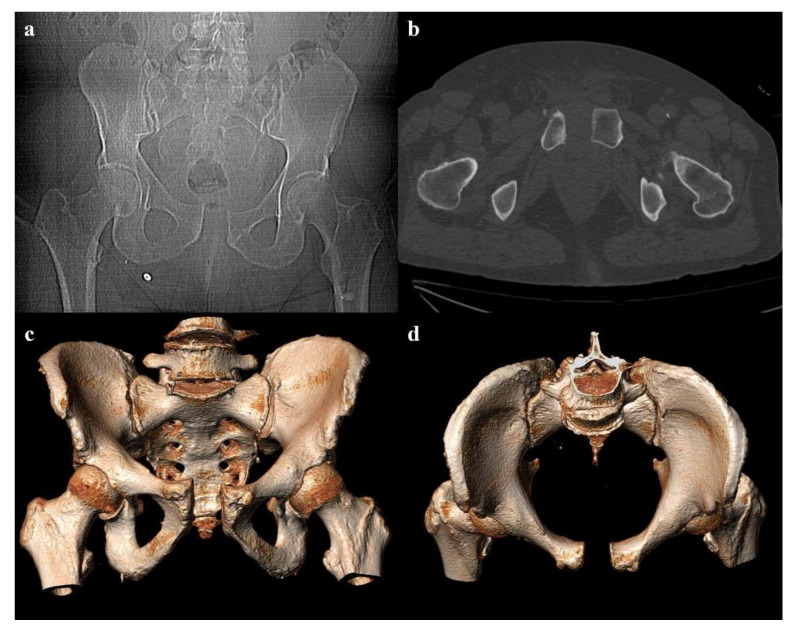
Anterior–posterior compression fracture, type 1. Anteroposterior scout CT view (a) and axial CT image (b) show a 2.4 cm diastasis of the pubic symphysis. Three-dimensional volume-rendering CT reconstructions in outlet and inlet views (c,d) well depict the diastasis.
